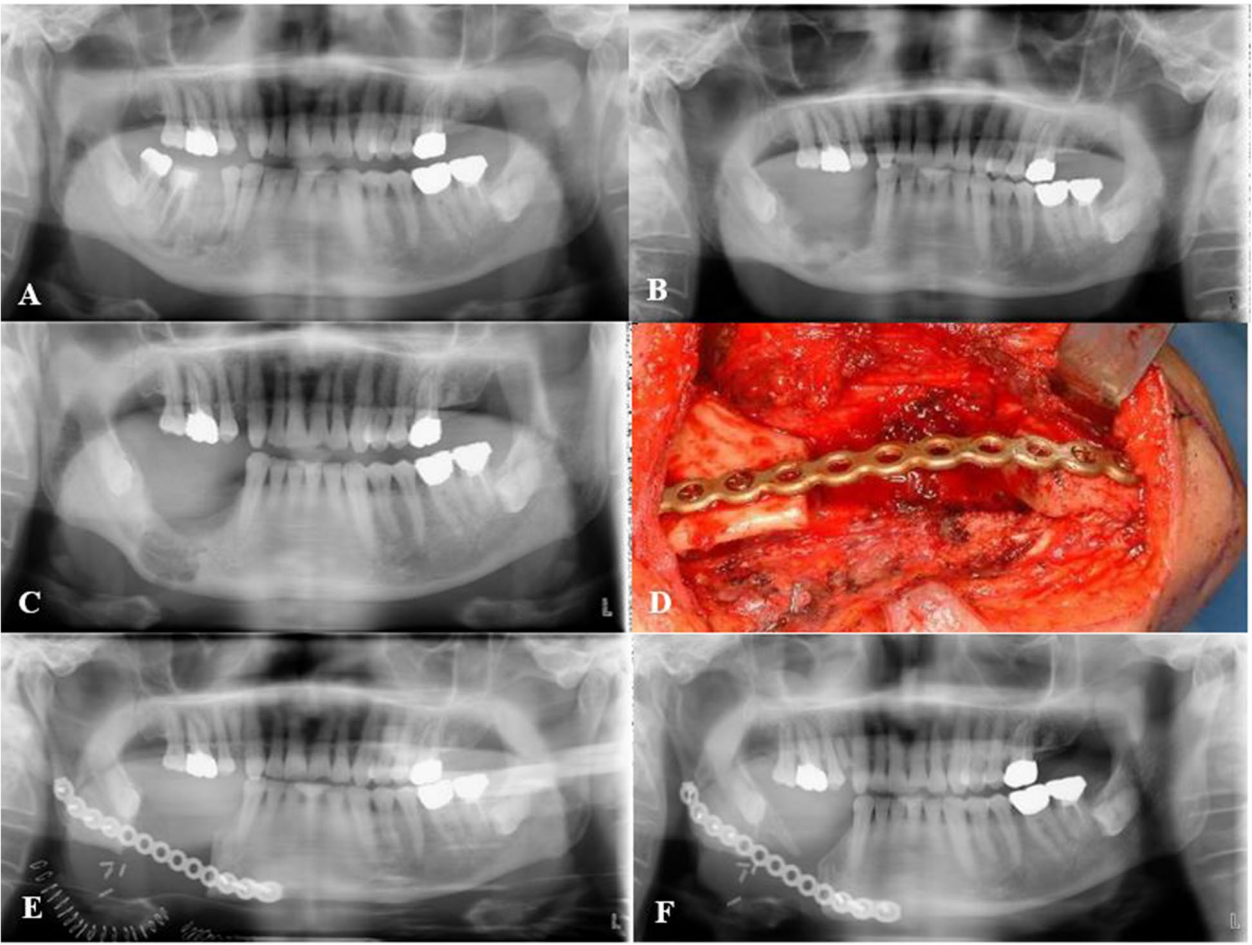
Surgical treatment of medication-related osteonecrosis of the jaw in prostate cancer patient. A Preoperative panoramic radiograph showing osteonecrosis of the right mandible in #45 area. B Postoperative panoramic radiograph after 2 weeks. C Postoperative panoramic radiograph after 1year showing the aggravation of the disease. D Partial mandibulectomy and reconstruction plate application. E Postoperative panoramic radiograph after 1day of mandibulectomy. F Postoperative panoramic radiograph after 1 year of mandibulectomy showing stable results
